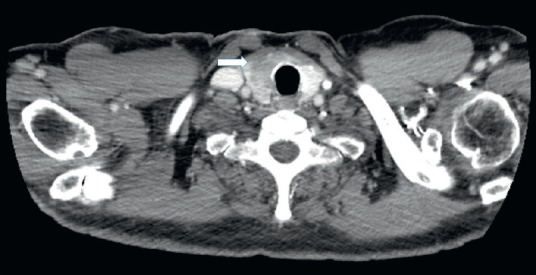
Axial CT scan after injection of contrast agent: 19 mm subtly hypodense nodule in the right thyroid lobe.
